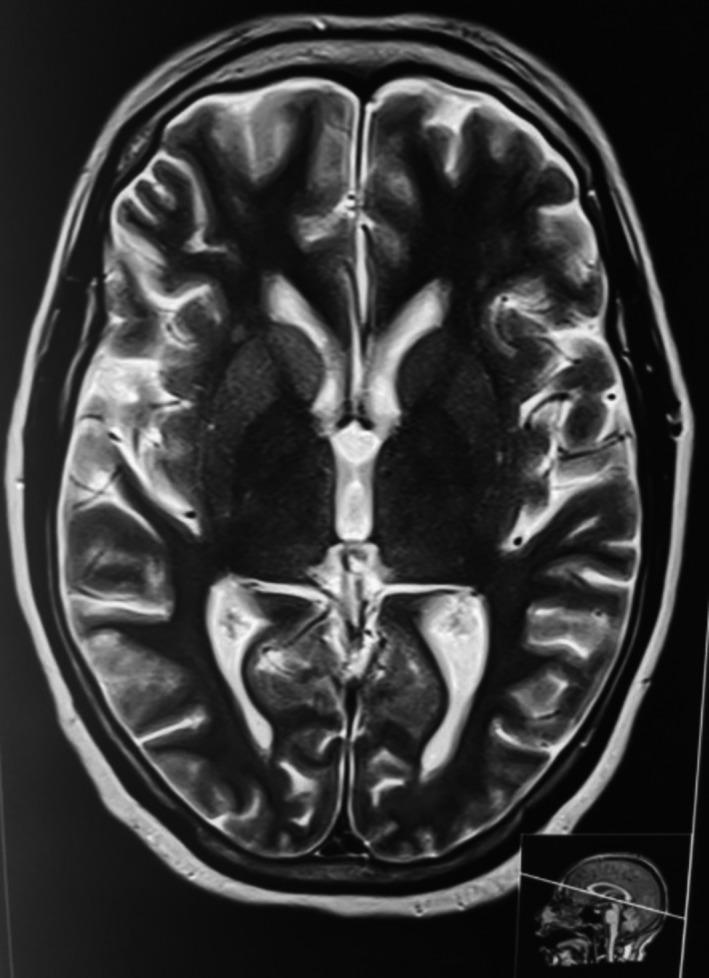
T2‐weighted MRI showing hyperintensity on right caudate and right lentiform nucleus
